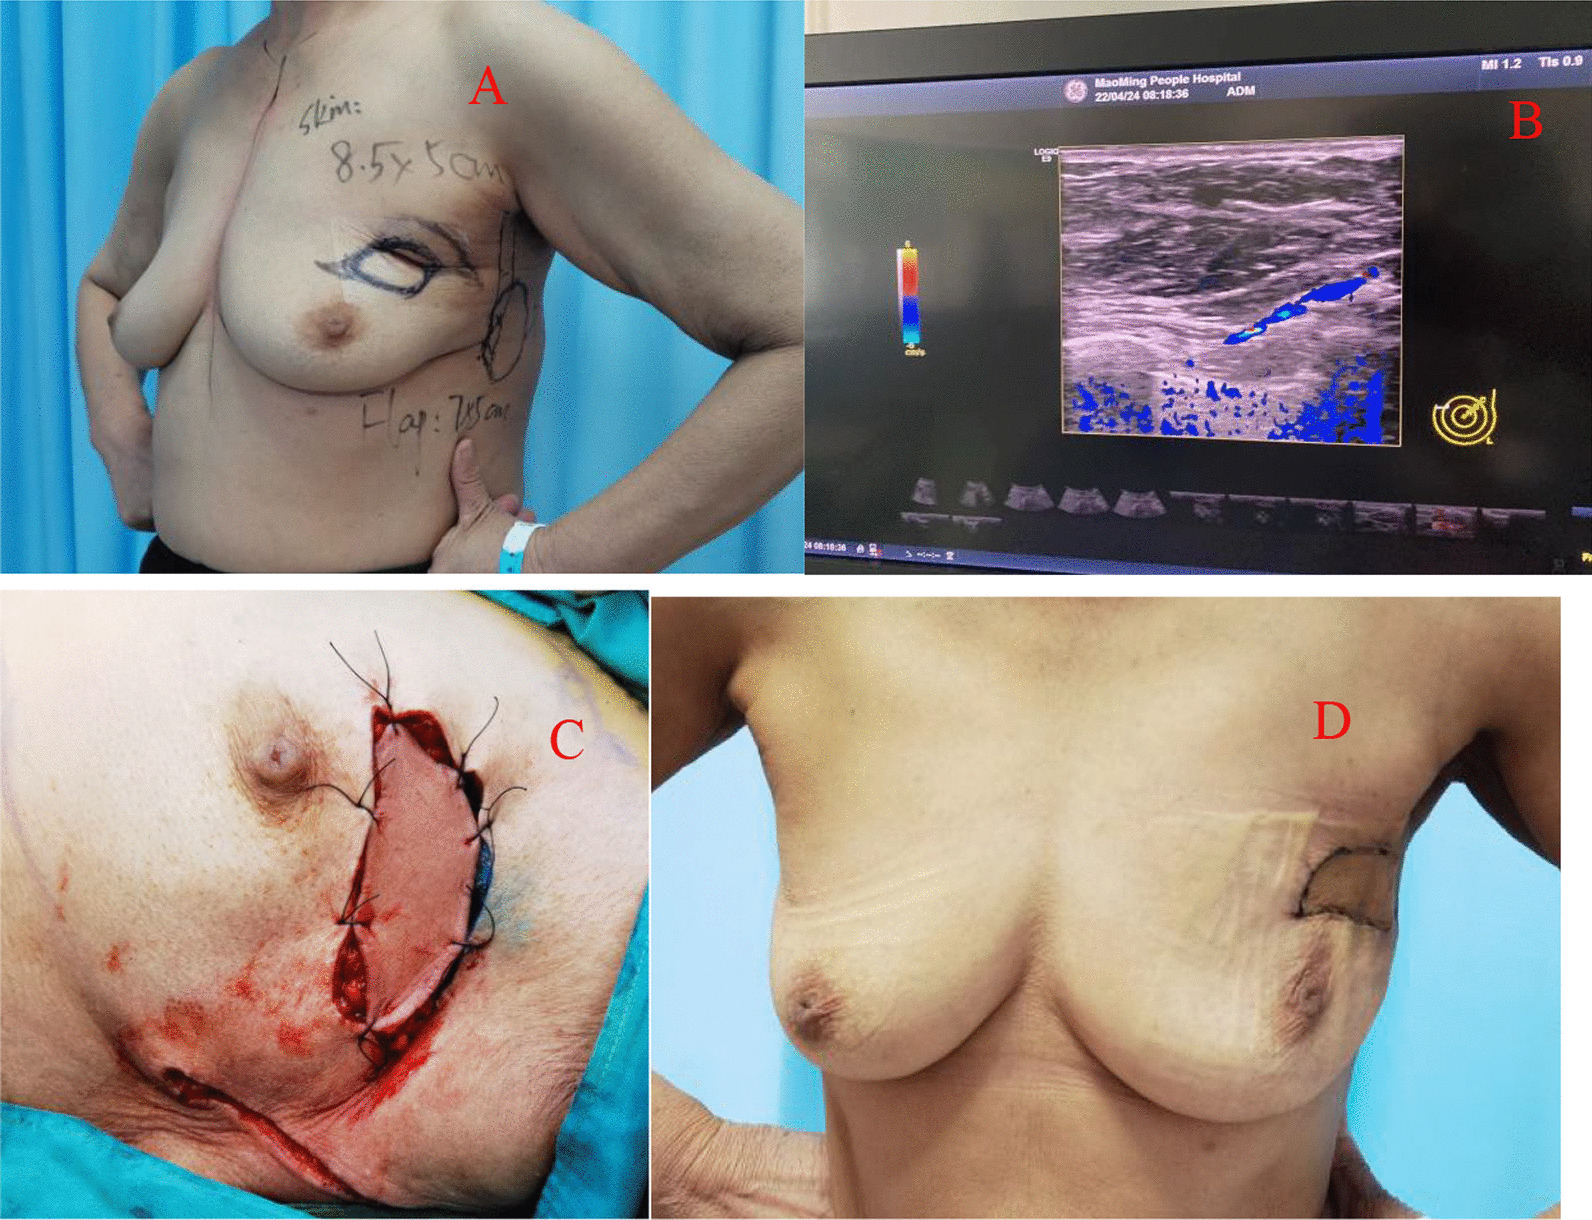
LICAP—A Designed resection plan and reconstruction flap preoperatively. B The 4th lateral intercostal artery perforator in a colour Doppler ultrasound image. C Filling the defect. D Three months after the operation
